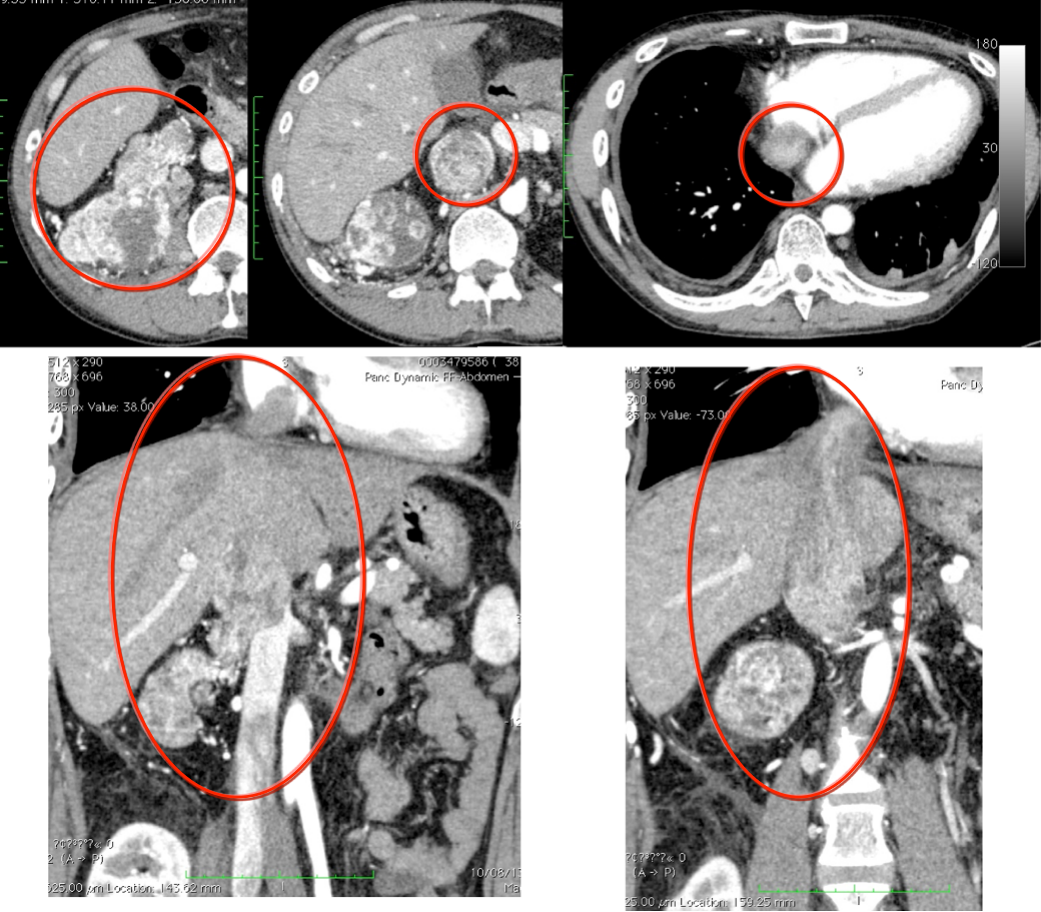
Computed tomography scan before preoperative sunitinib therapy. The level of tumor thrombus was evaluated as IV according to Novic’s classification. The red circle indicates the tumor thrombus.
